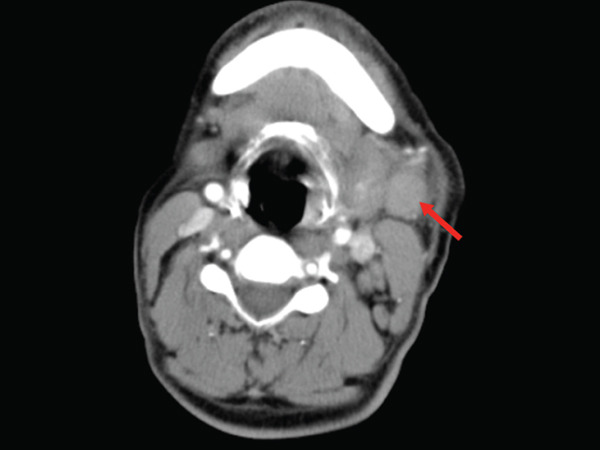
Axial slice of CT‐enhanced scanning of the lesion.
